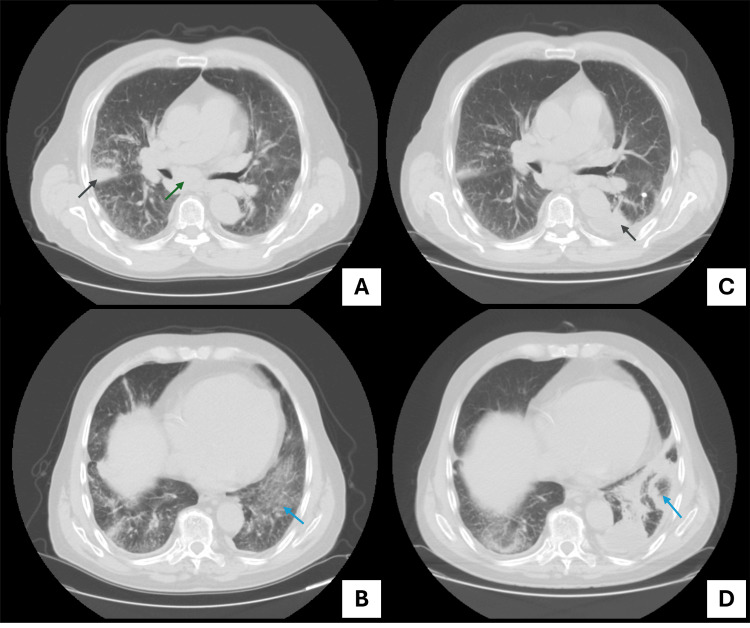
Evolution of radiologic findings on CT scan. Single-level views from the follow-up CT scan performed after the first hospitalization (A and B) and the CT scan obtained during the second hospitalization (C and D). (A) Focal lung consolidation (dark blue arrow) and enlarged mediastinal lymphadenopathy (green arrow). (B) Bilateral ground-glass opacities, more pronounced in the left lower lobe (light blue arrow). (C) Findings similar to those observed in Panel A, with the addition of a new left lower lobe consolidation (dark blue arrow). (D) A left lower lobe consolidation accompanied by fibrotic bands and a radiologic atoll sign, defined as a central focus of ground-glass opacity surrounded by a peripheral ring of consolidation (light blue arrow).
